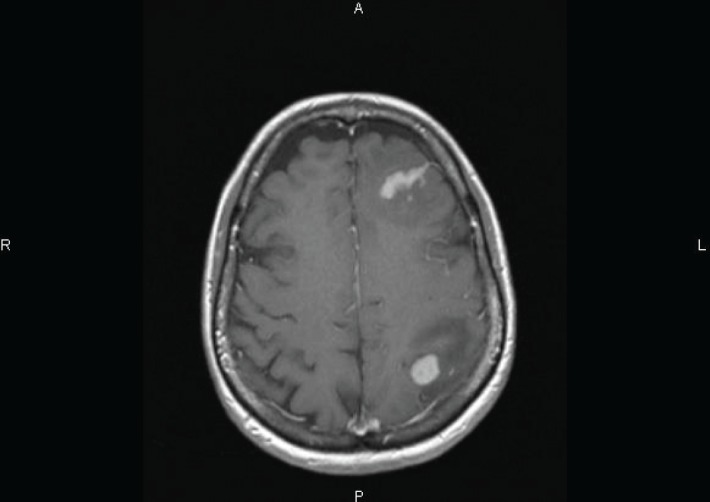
Axial T1-weighted MRI image performed after Gadolinium injection showing two enhancing lesions surrounded by oedema, located in the left frontal and frontoparietal regions.
